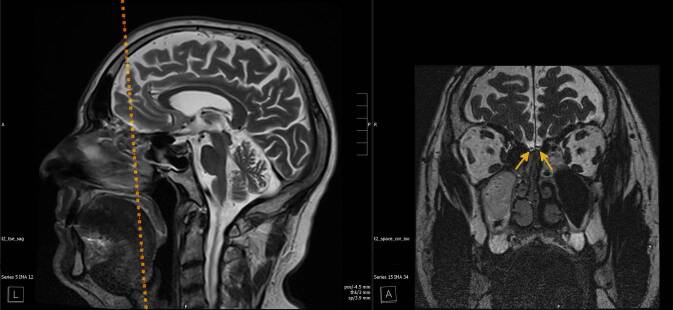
Beispielhafte Ausrichtung der koronalen Rekonstruktionen anhand einer T2-gewichteten („T2-weighted“, T2w) Turbospinecho(TSE)-Sequenz sagittal (*gestrichelte Linie*: Ebene der koronalen Rekonstruktion aus der T2w-„sampling perfection with application-optimized contrast using different flip angle evolution“[SPACE]-Sequenz [*rechts*], *Pfeile* Bulbi olfactorii)
